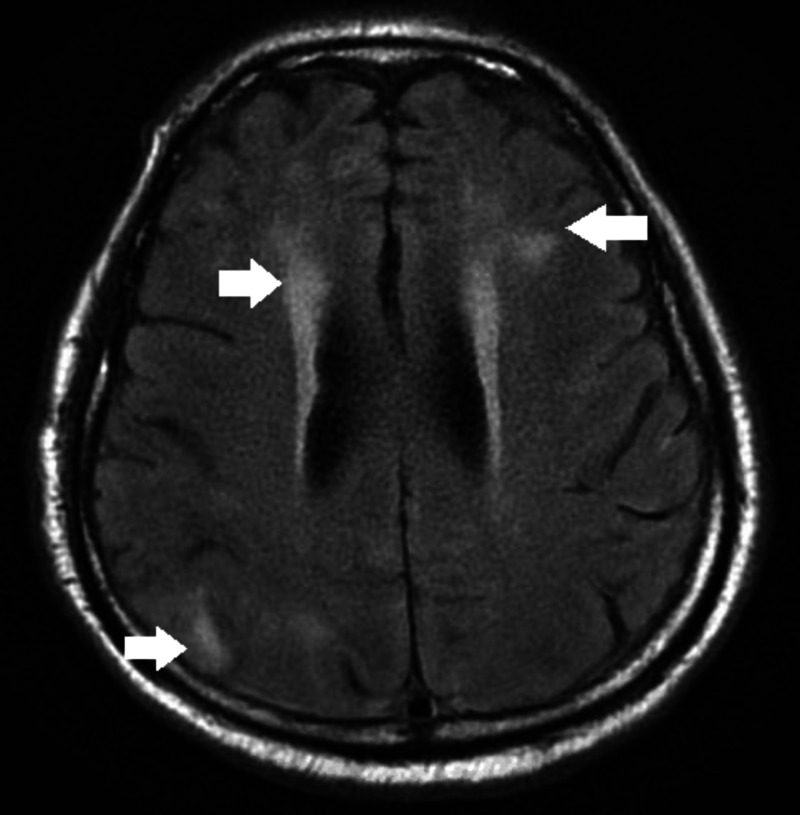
Magnetic resonance imaging (MRI) brain without contrast showing lateral periventricular and right parieto-occipital bright signals concerning for cerebritis
